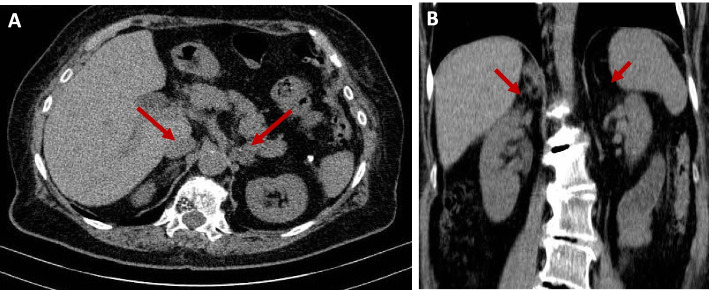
Follow-up Computed tomography scan of the abdomen and pelvis of the second case after three months showed a stable size and decreasing density on both sides with a mean HU < 10 in non-contrast images. (A: axial view and B: Coronal view). The red arrows indicate adrenal hemorrhage
